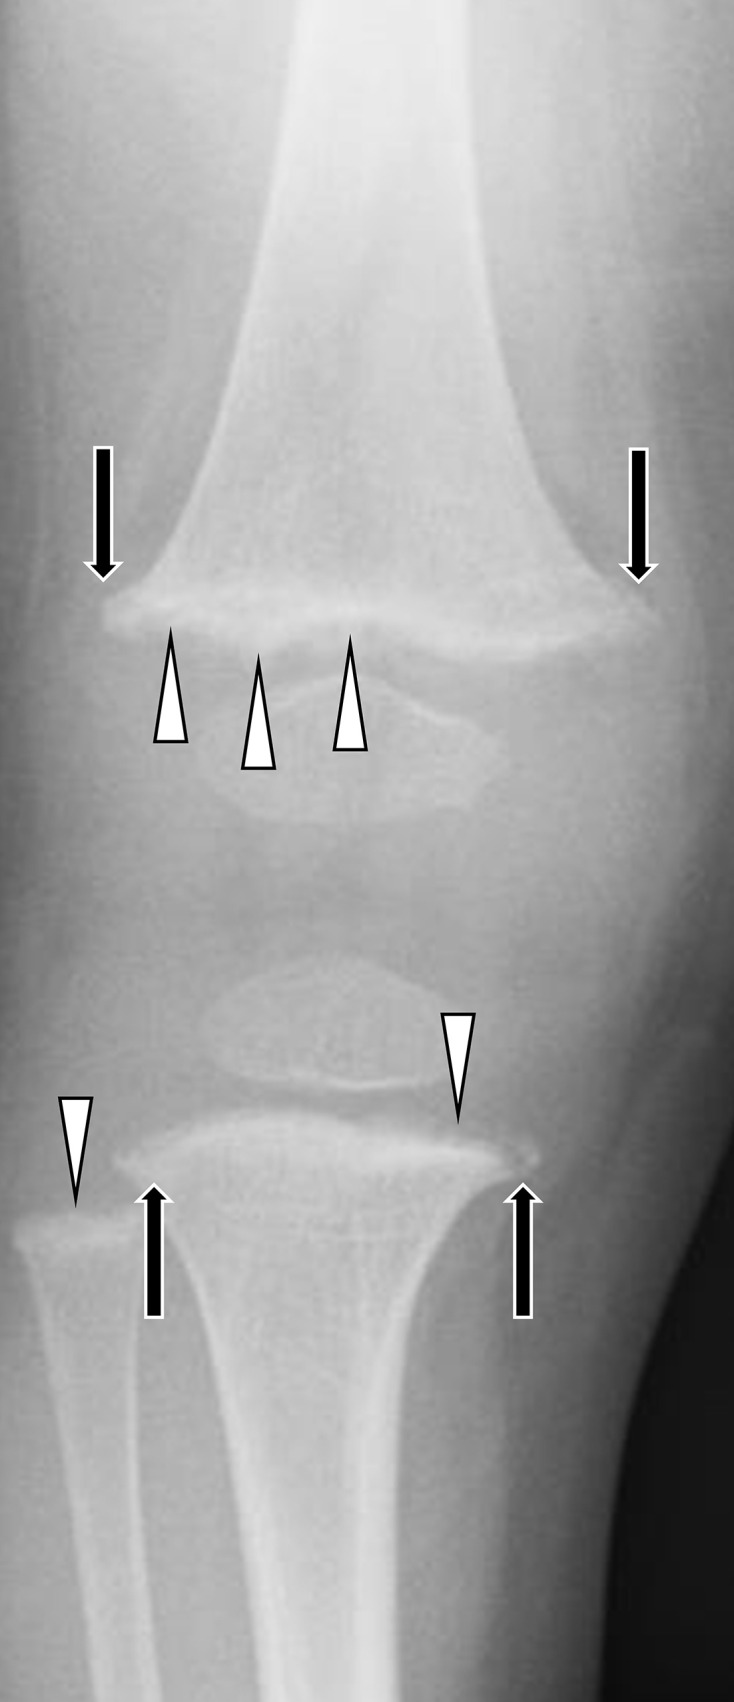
Rickets in the knee. An 18-month-old boy who was exclusively breastfed lost his appetite after starting nursery school. His mother was aware of the difference of the length of his lower extremities. Posteroanterior radiograph demonstrated indistinct metaphyseal margins in the femur, tibia and fibula (fraying; arrowheads) and the widening of metaphyseal ends in the femur and tibia (splaying; black arrows). Healing stage was suggested due to the provisional zone of calcification although actual onset was unknown. His activated vitamin D level (0.87 pg ml^−1^) was lower than the paediatric normal range (20–70 pg ml^−1^).
